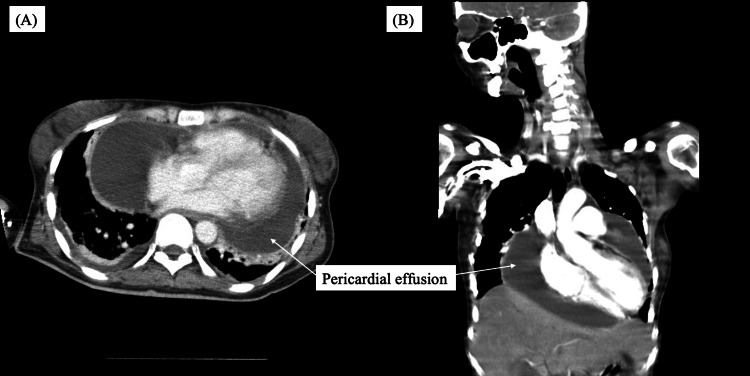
Chest computed tomography on day 0 showing pericardial effusion (A) Axial view showing circumferential pericardial effusion. (B) Coronal view confirming extensive pericardial effusion compressing the heart.
